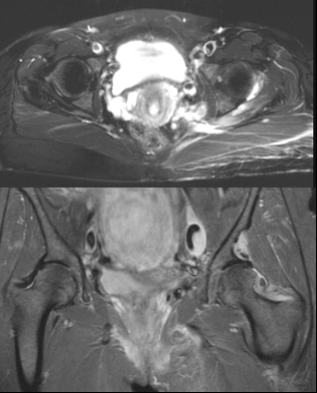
Transverse and Coronal MRI views demonstrating neurofibroma above left femoral neck.
